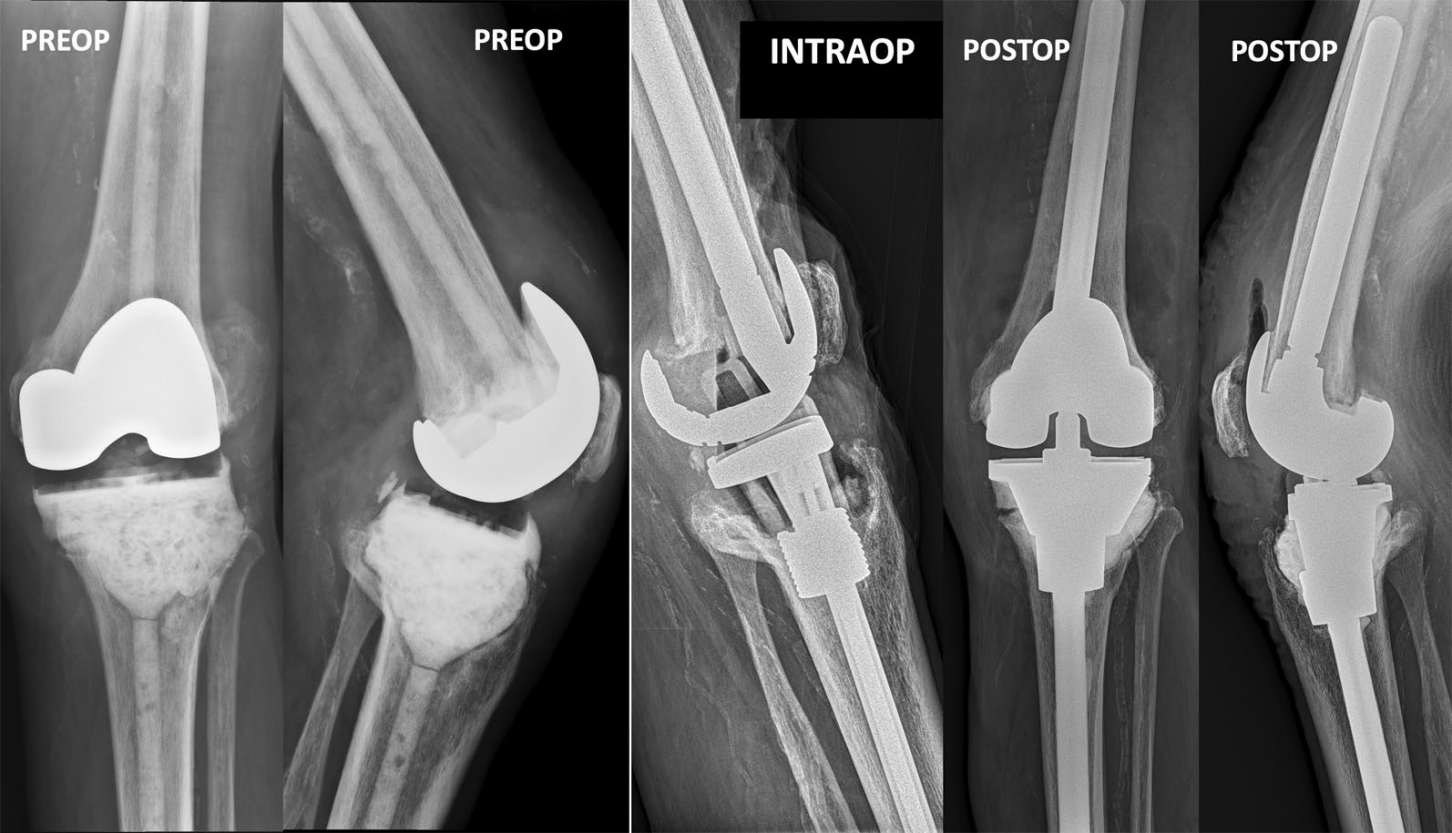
Seventy-eight-year-old patient with a right knee dynamic spacer following a periprosthetic joint infection (PJI). Left: preoperative anteroposterior and lateral radiographs. Center: intraoperative lateral radiograph showing alignment of the trial components and amount of bone loss (Anderson Orthopaedic Research Institute Knee Bone Loss Classification—AORI 3) [6]. Right: postoperative anteroposterior and lateral radiographs, showing stacked trabecular metal cones (small and large) on the tibia
